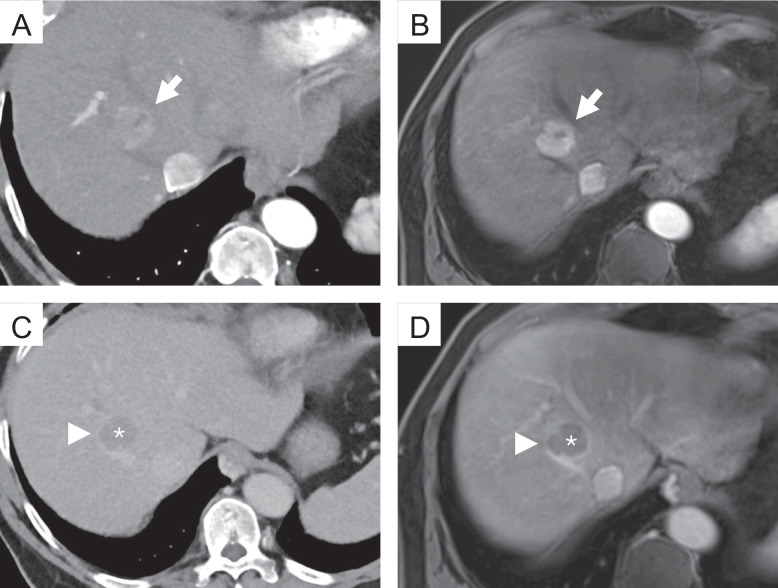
Examples of the different major features of the LI-RADS classification. Top row: Photon-counting detector computed tomography (PCD-CT) (A) and magnetic resonance imaging (MRI) (B), hepatic arterial phase; the lesion shows strong contrast enhancement compared to the surrounding liver parenchyma (non-rim arterial phase hyperenhancement, APHE) (arrow). Bottom row: PCD-CT (C) and MRI (D), delayed phase; the lesion shows a reduction in enhancement relative to the surrounding background liver (non-peripheral “washout”) (*). Note the smooth border around the largest parts of the observation (enhancing “capsule” (arrowhead))
